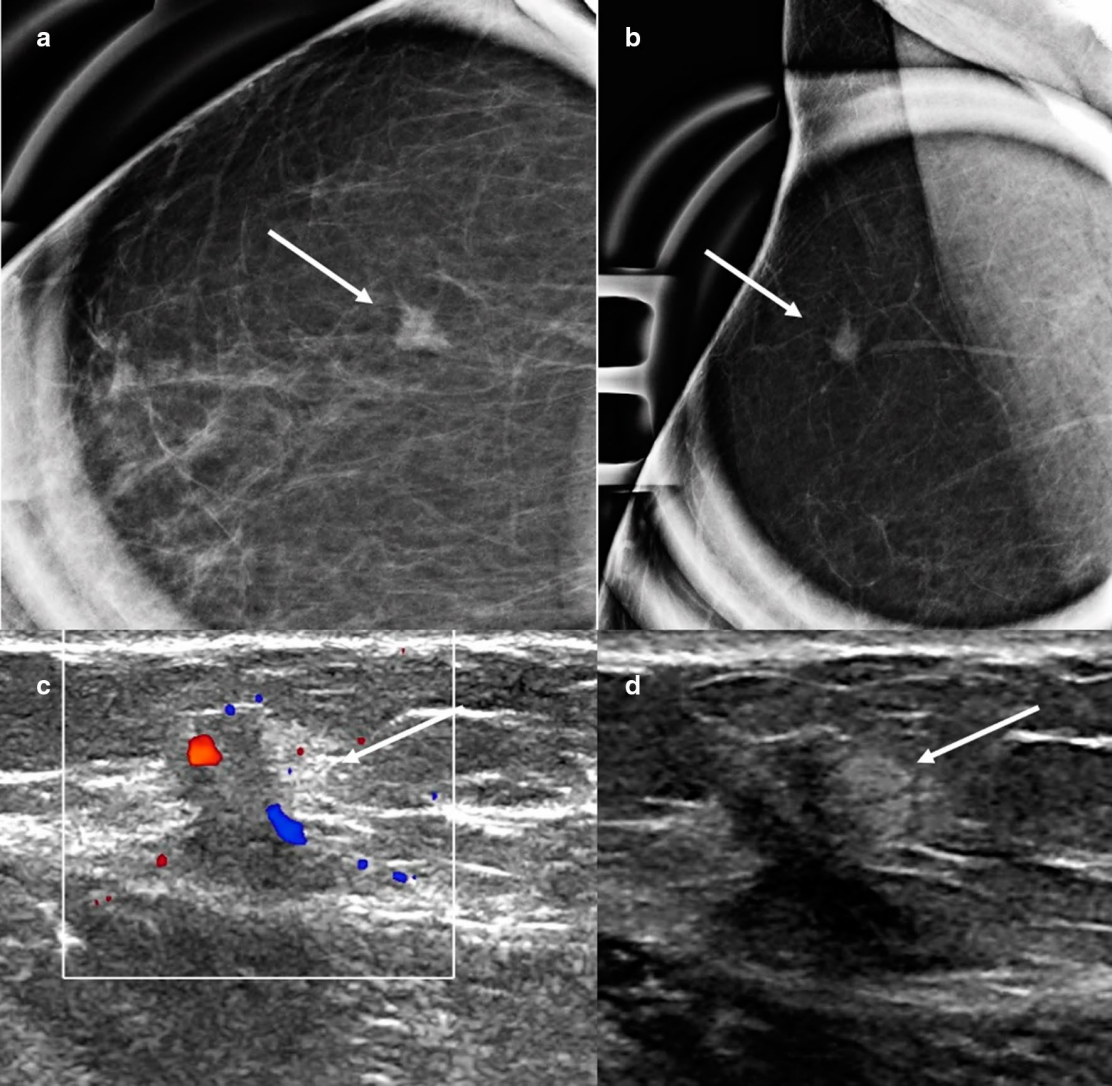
Granular cell tumor. A 65-year-old woman presented for further evaluation of a focal asymmetry detected on screening mammopgrahy. Craniocaudal and lateral spot compression views (a, b) show a small irregular mass with spiculated margins (arrows). Color Doppler (c) and grayscale (d) ultrasound shows a corresponding irregular mass with internal vascularity (arrows). Core needle biopsy yielded granular cell tumor which was treated with surgical excision
